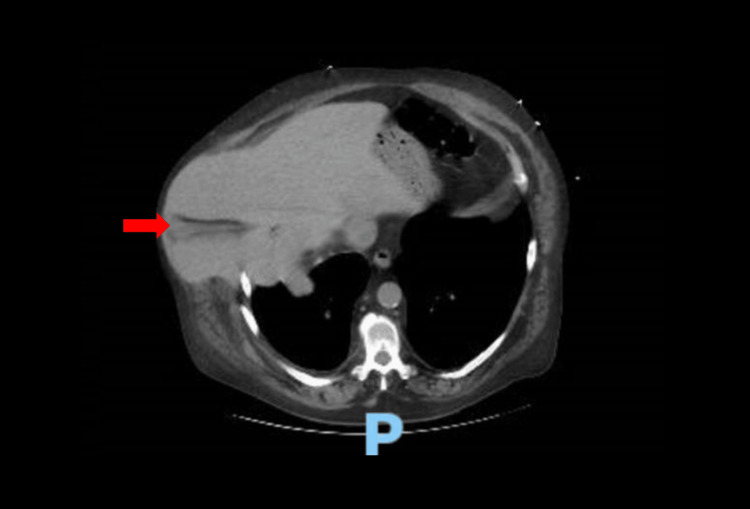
Axial computed tomography image showing right liver lobe herniation Red arrow: Liver herniation
